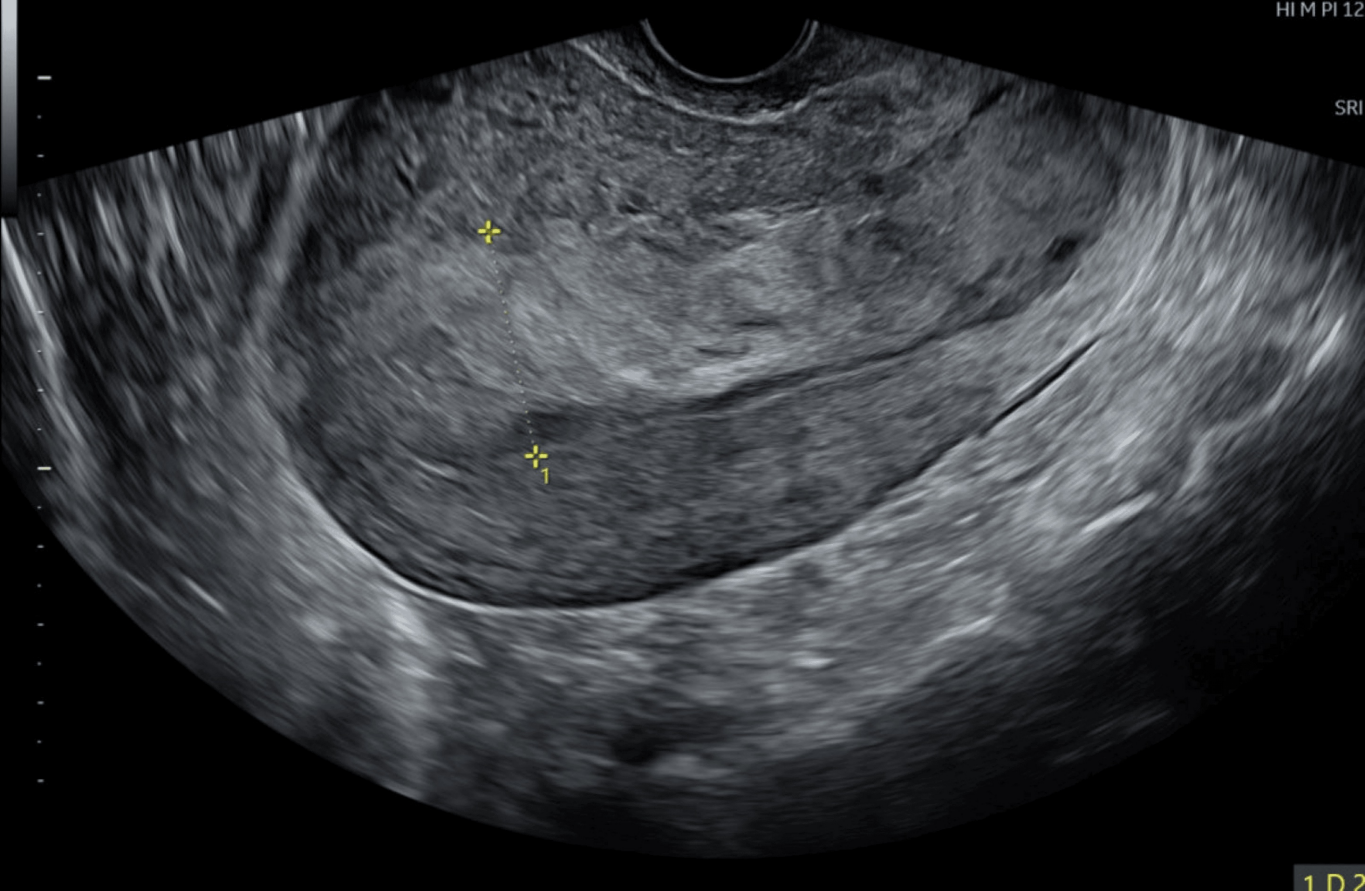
Ultrasound scan image showing the endometrial thickness of 29 mm.
